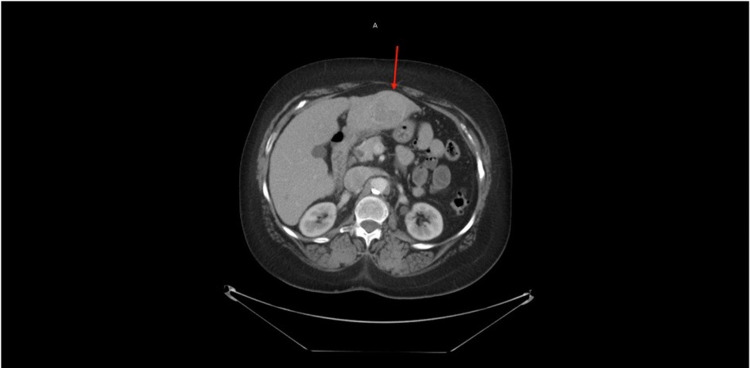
CT chest without contrast showing left hepatic lobe lesion with heterogeneous enhancement.
